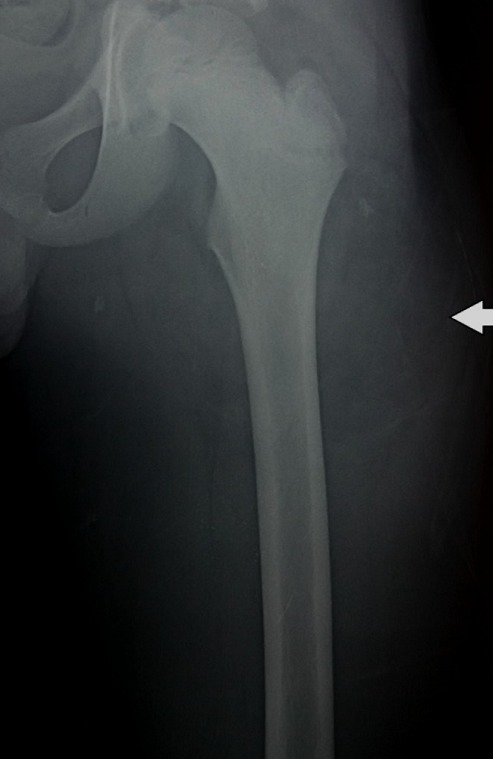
Radiographie standard de la cuisse gauche montrant une opacité des parties molles latérales externes sans anomalie osseuse en regard
